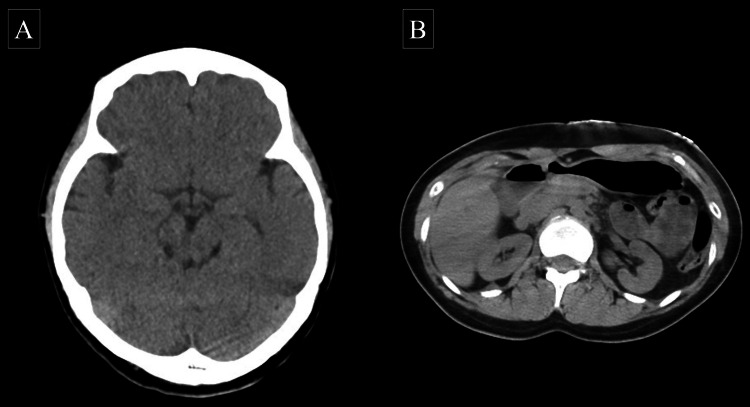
Head (A) and abdomen (B) CT images prior to the start of ECMO in case 2. The CT shows no trauma findings in the head and trunk. ECMO, extracorporeal membrane oxygenation; CT, computed tomography
